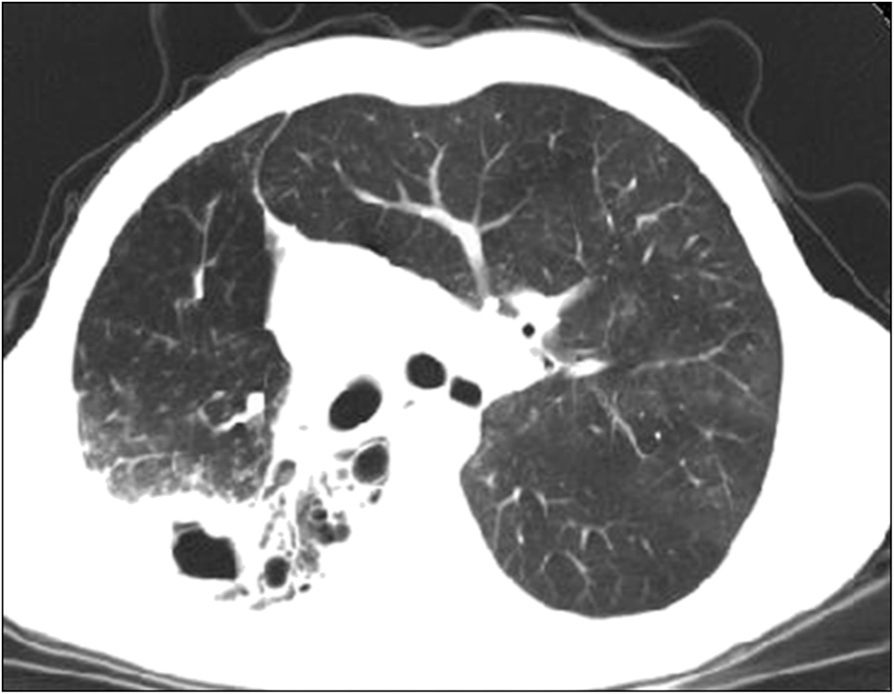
CT showing cavitation and infiltration.
